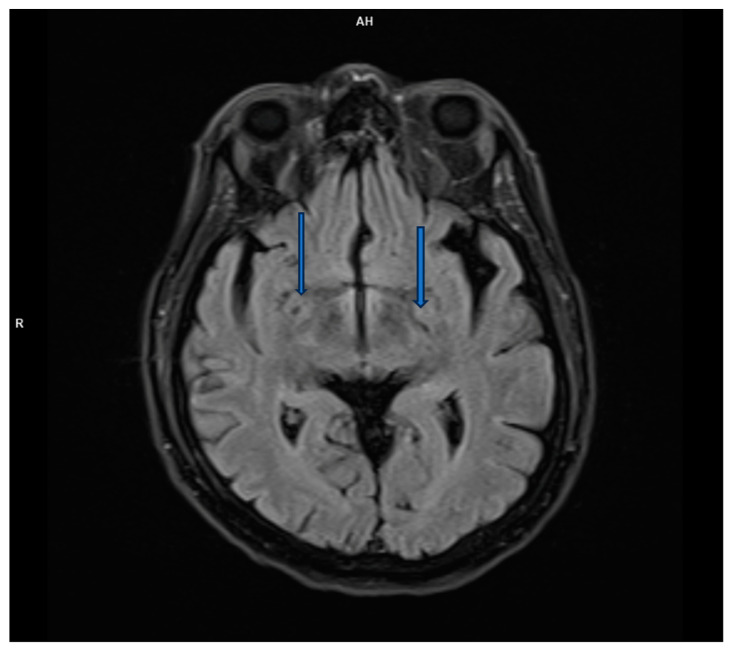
FLAIR transverse MRI scan demonstrates prominent perivascular spaces.
